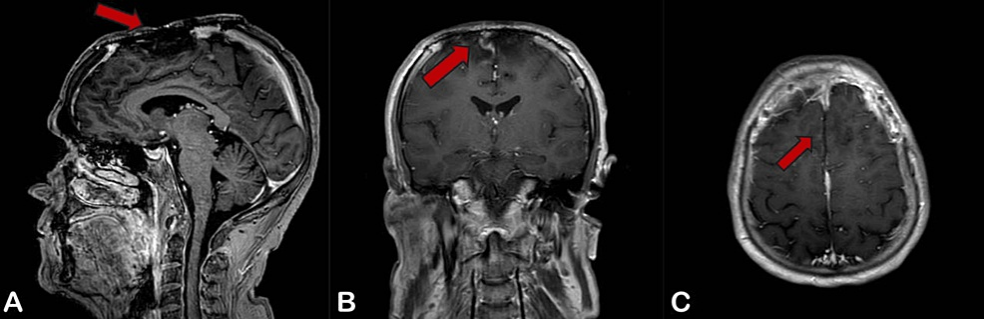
(A) Sagittal, (B) Coronal, and (C) Axial T2-Weighted MRI With Contrast, Showing Post-frontoparietal Cranioplasty for Meningioma Resection With Bilateral Frontal Encephalomalacia, Gliosis, and Small Left Parafalcine Residual Lesions.
